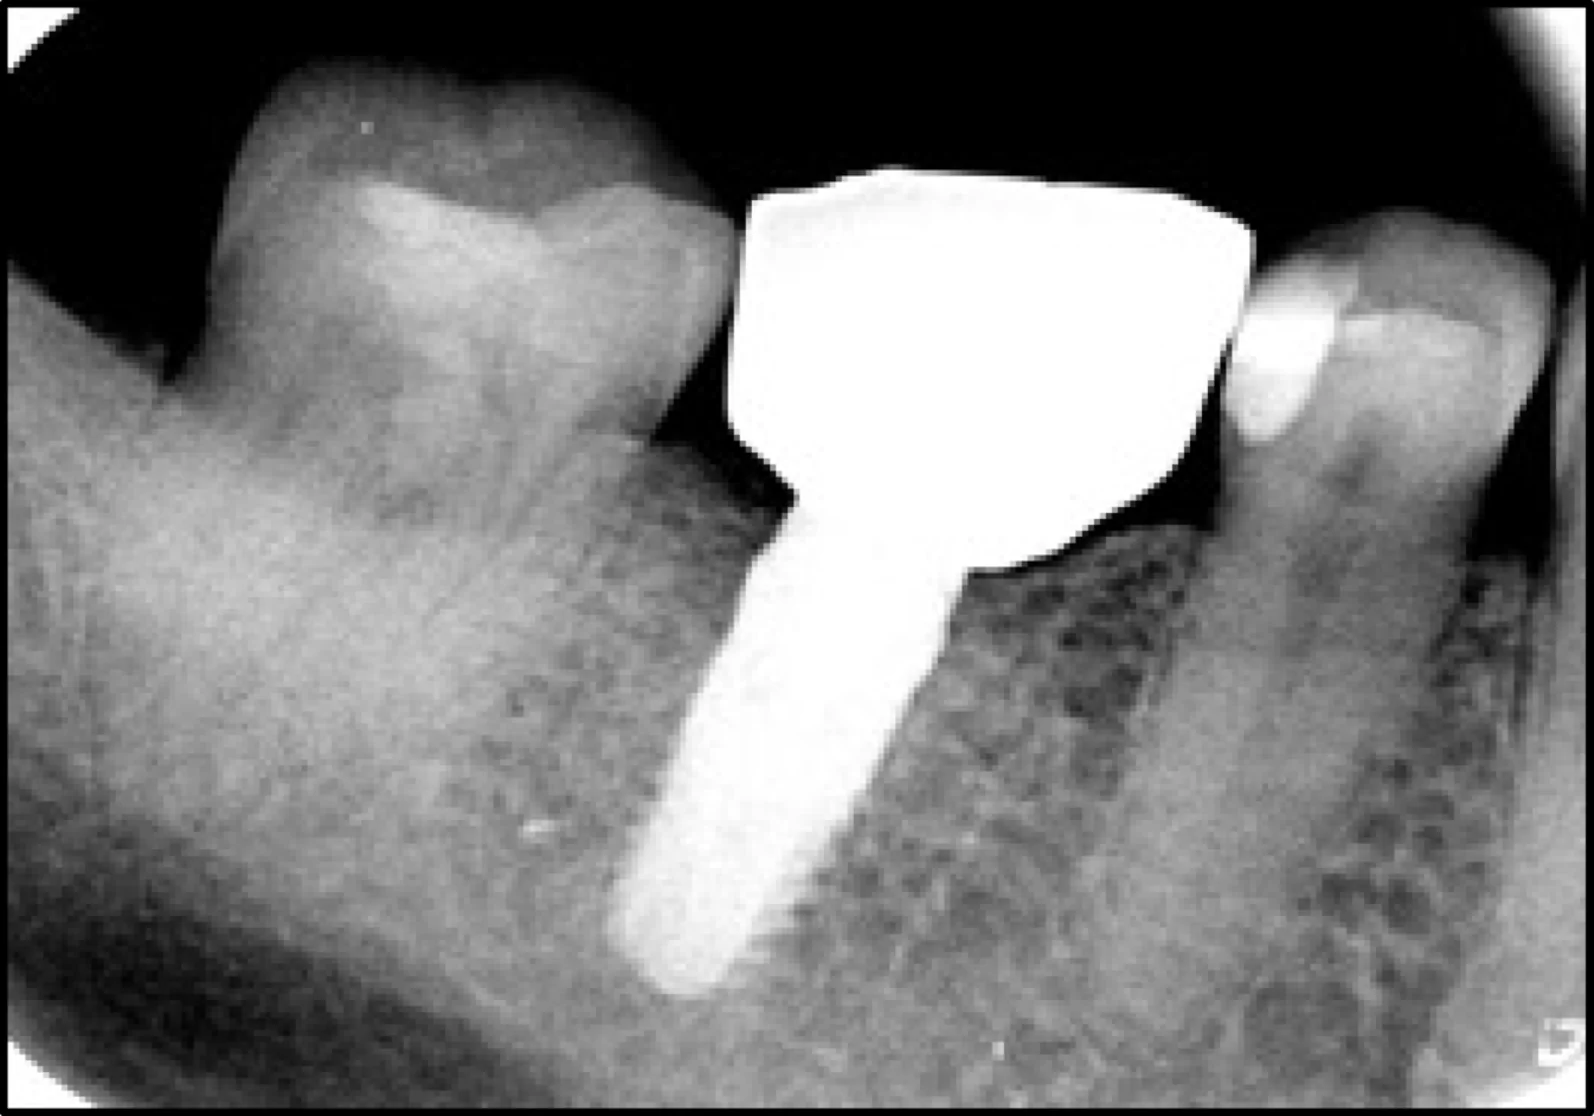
Radiographic confirmation of complete seating of the crown–coping complex on the conometric abutment
